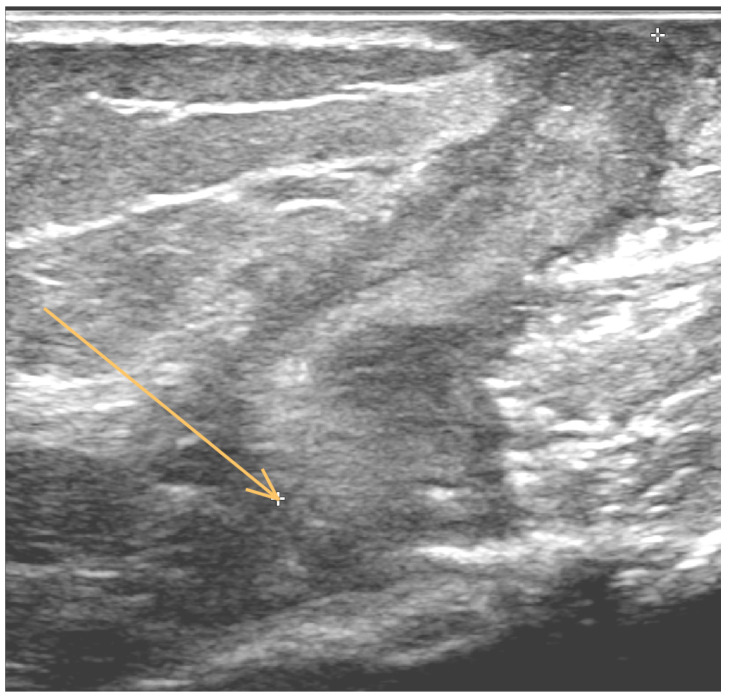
Ultrasound examination of the proximal part of the right arm shows a fluid-thickened collection (indicated by arrow).
